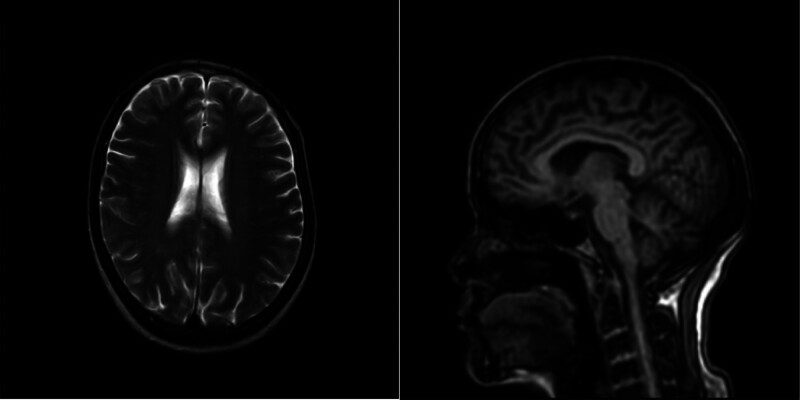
Brain MRI: bilateral cerebral hemispheres had a little ischemic degeneration.
